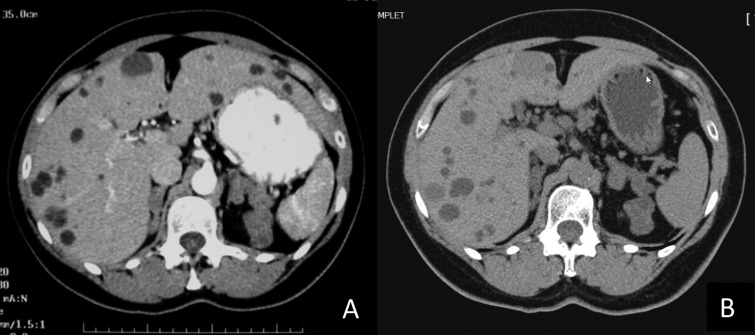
Abdominal CT-scan at baseline (A) and after 10 years on low-dose ketoconazole (B). Multi-lobulated bilateral adrenal masses are observed, which remain unchanged in morphology after 10 years of treatment. Volume was calculated using the ellipsoid formula π abc/6 (ml): (a) anterior–posterior, (b) transversal, and (c) cranial–caudal maximal diameters. (A) Right adrenal 12.9 ml and left adrenal 20.5 ml. (B) Right adrenal 14.7 ml and left adrenal 20.9 ml. Hepatic cysts are due to familial polycystic liver disease.
